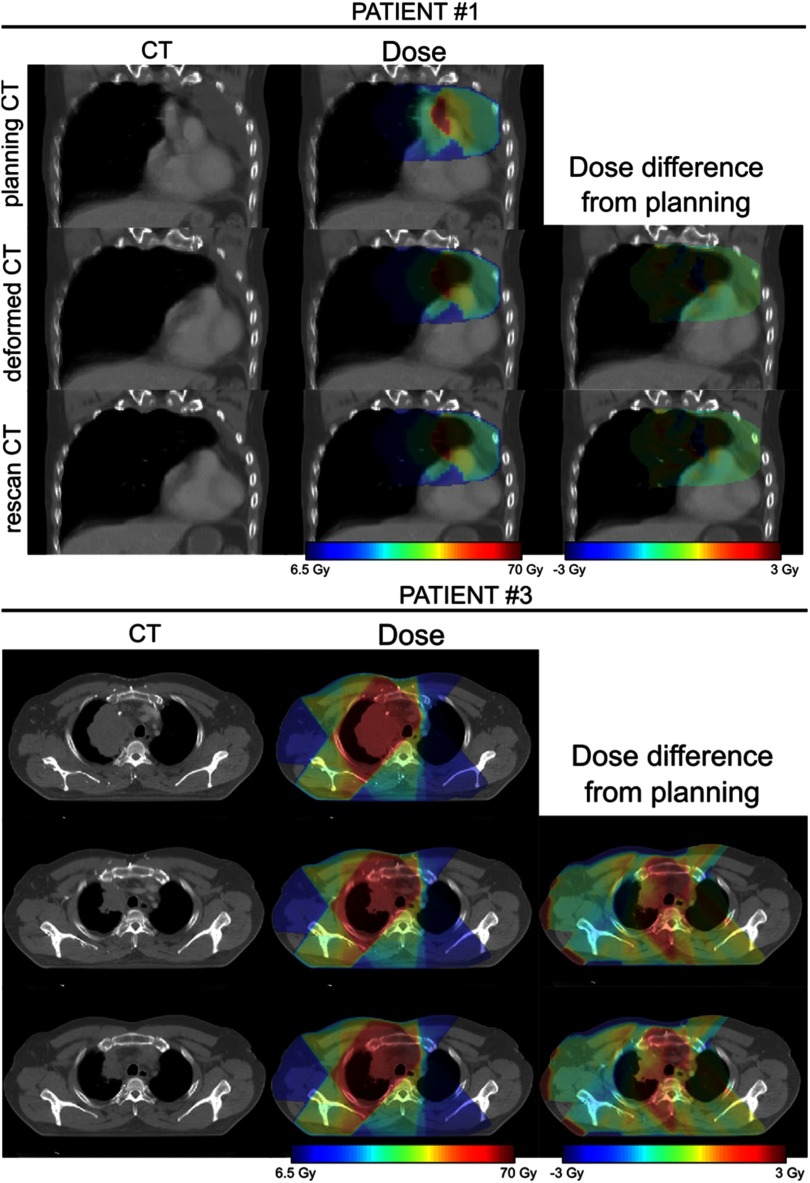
Comparison of rCT and dCT CT scans and dose distributions (against pCT) for two of the patients included in the study. In each case the prescription dose was 64 Gy.
